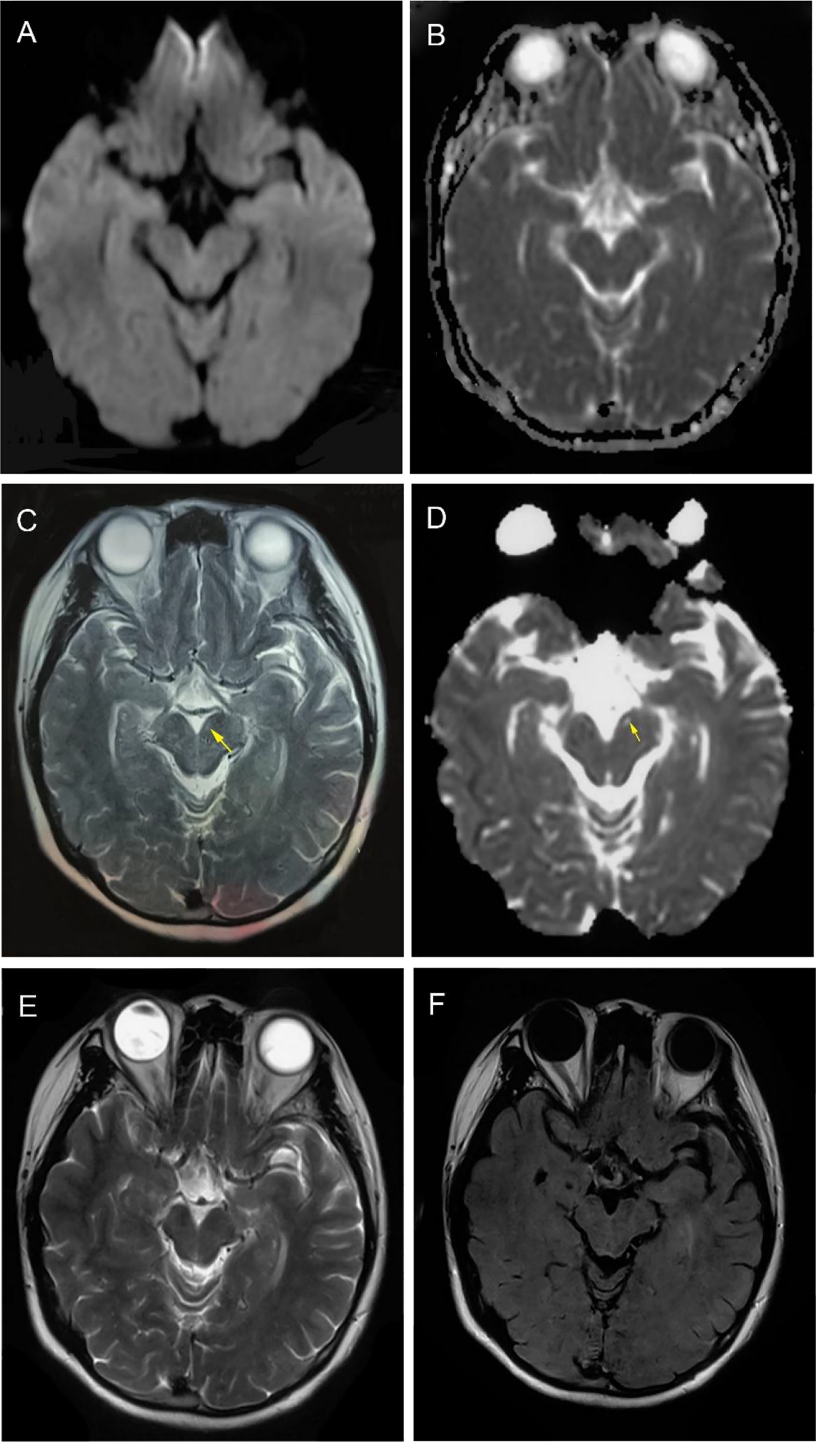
Magnetic resonance imaging findings. At time of diagnosis, brain MRI DWI and ADC sequences (transverse) showed no suspicious signal alterations (A, B). Four months after onset, T2 and ADC sequences showed obvious high signals on the left midbrain interpeduncular fossa (highlighted by arrows) (C, D). One year later, T2 and T2-flair sequences (transverse) showed midbrain lesions disappeared. (E, F).
